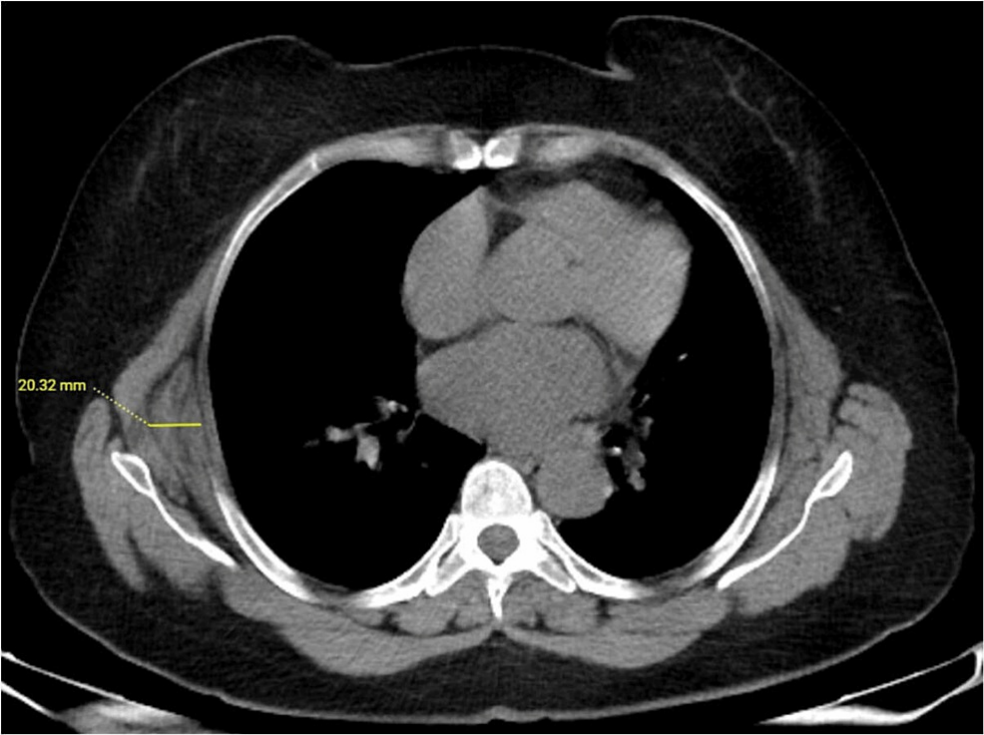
Axial non-contrast CT image of a 65-year-old female patient with right unilateral ED. The thickness measurement of ED was made by measuring the widest diameter on the axial image.
